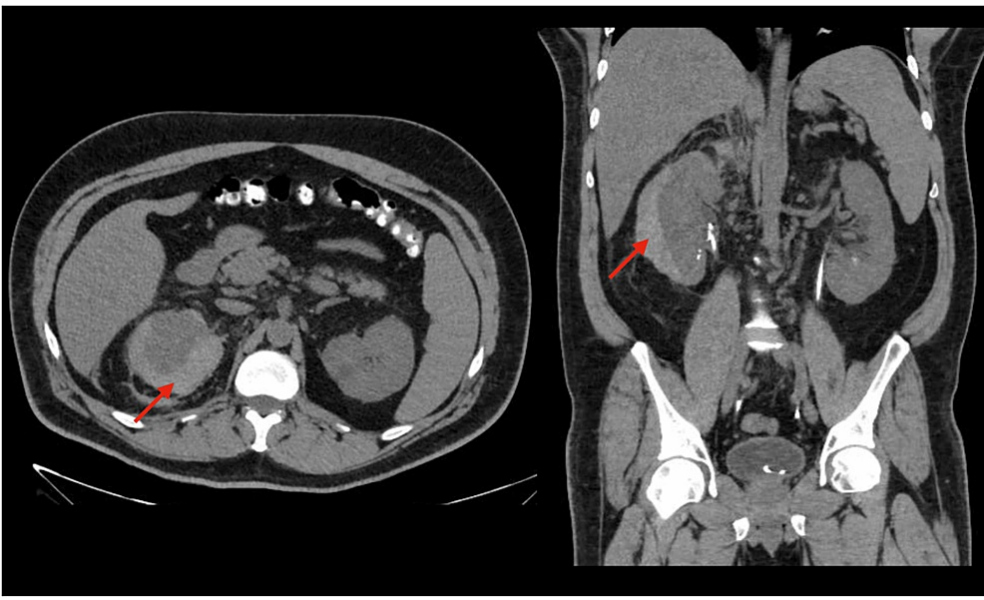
Axial and coronal views of a CT of the abdomen without contrast shown at the level of the kidneys The red arrows point toward a hyperdense lesion delimiting the renal capsule, measuring 50 HU, compatible with a perinephric hematoma. CT: computed tomography, HU: Hounsfield units
